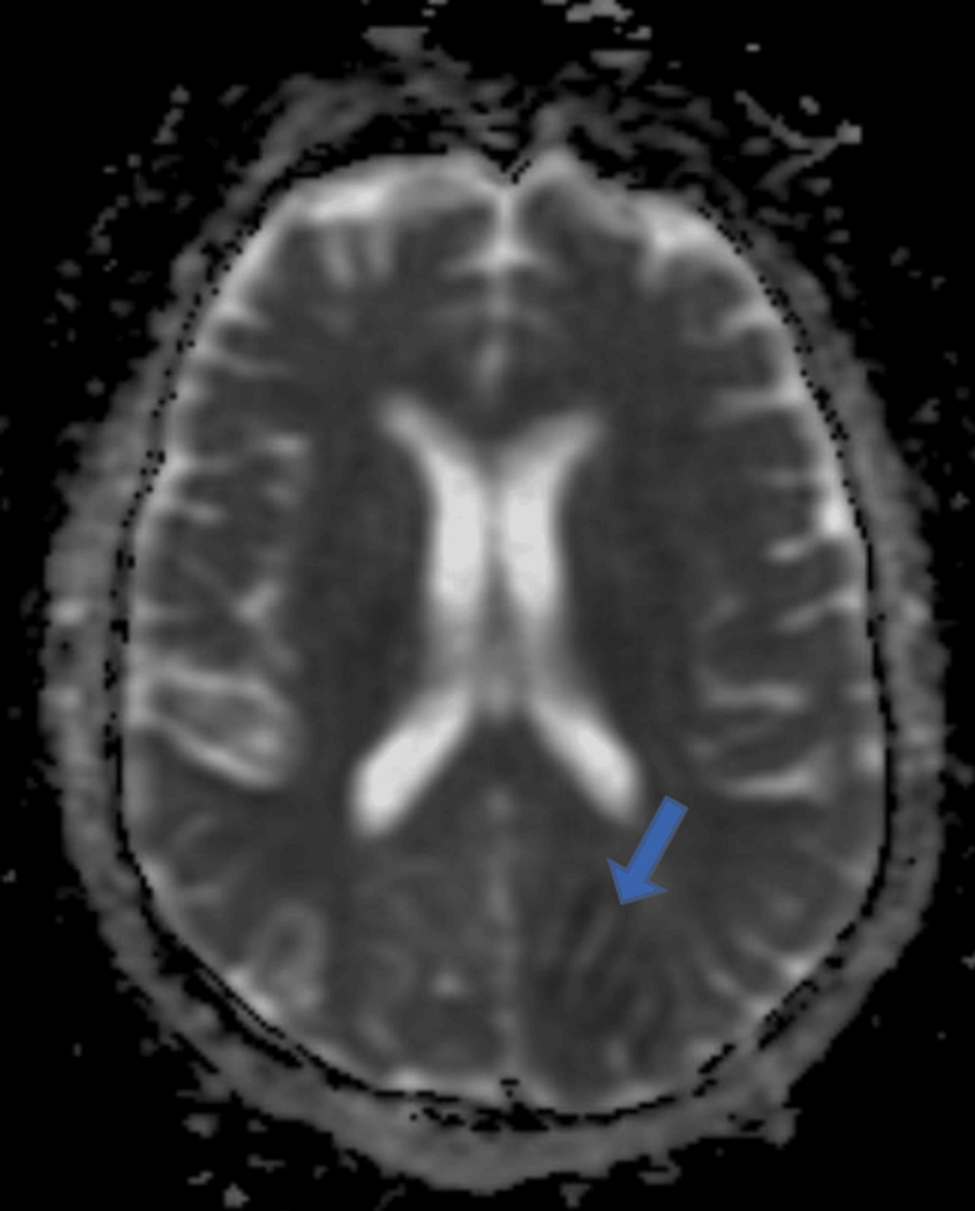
MRI brain showing few areas of diffusion restriction which were of low intensity on the ADC map on the left side with gyriform pattern ADC: apparent diffusion coefficient
